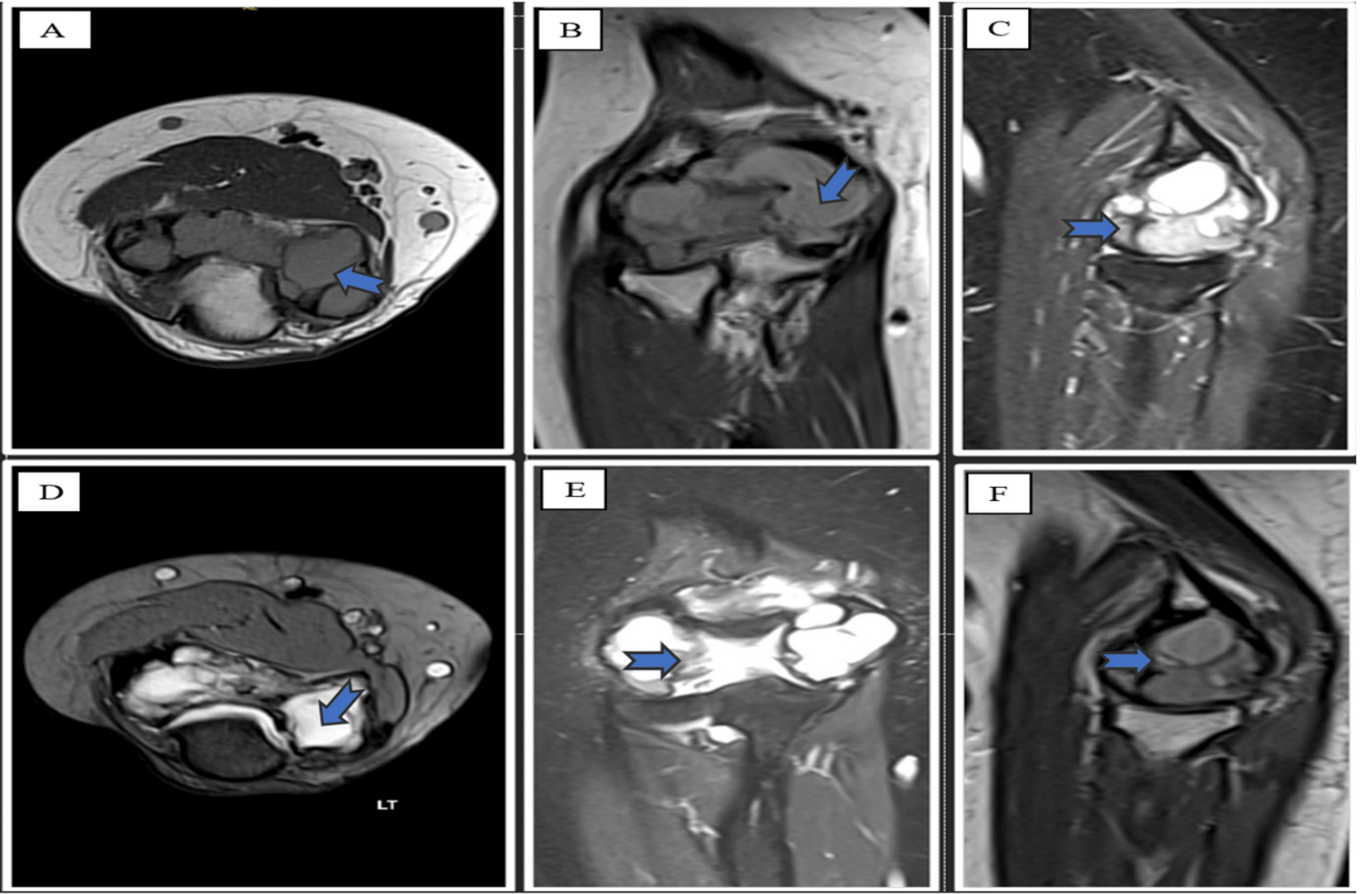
MRI demonstrated a large, eccentric, solid, and cystic lesion at the epiphysis of the left distal humerus, causing cortical breakthrough. (A) An MRI T1 axial view of the elbow. (B) An MRI T1 coronal view of the elbow. (C) The elbow's MRI T1 sagittal view. (D) An MRI T2 axial view of the elbow. (E) An MRI T2 coronal view of the elbow. (F) An MRI T2 sagittal view of the elbow. MRI: magnetic resonance imaging
